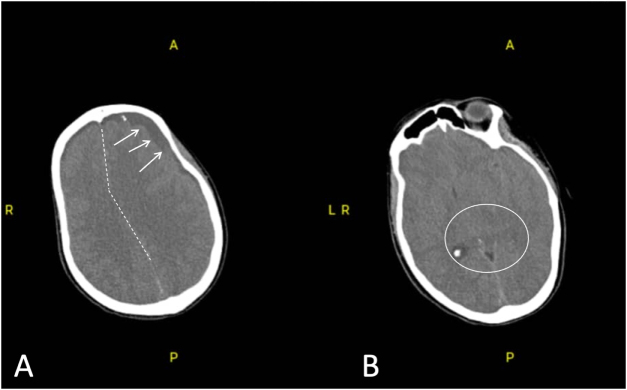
Preoperative non-contrast cranial CT. (A) A non-contrast axial CT scan demonstrates a large left frontoparietal subacute subdural hematoma with mixed-density (predominantly hypodense) components, measuring approximately 20 mm in maximal thickness (arrows). There is a significant mass effect with effacement of the left convexity sulci, compression of the left lateral ventricle, and a rightward midline shift of approximately 12 mm (dashed line). (B) A more posterior axial section again shows the left-sided subacute subdural hematoma with persistent mass effect and rightward midline shift. The basal cisterns appear compressed (encircled), consistent with early transtentorial herniation. All images were fully anonymized.
